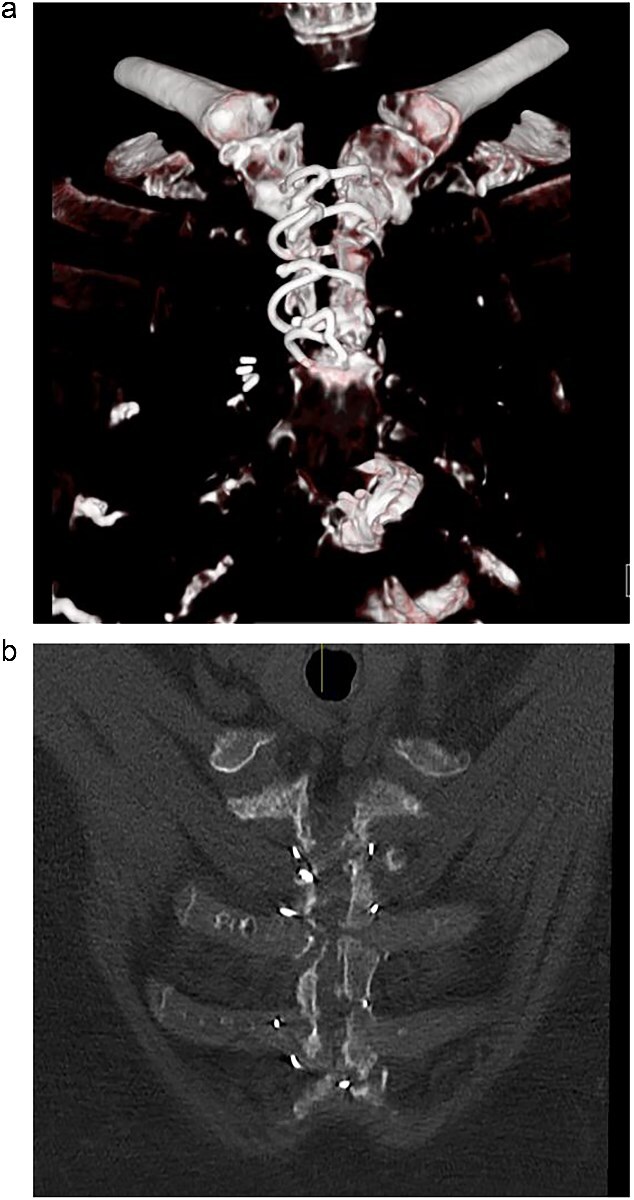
(a) Pre-operative CT scan showing dehisced upper hemi-sternum. (b) Pre-operative CT showing significant bony loss on the right hemi-sternum.
